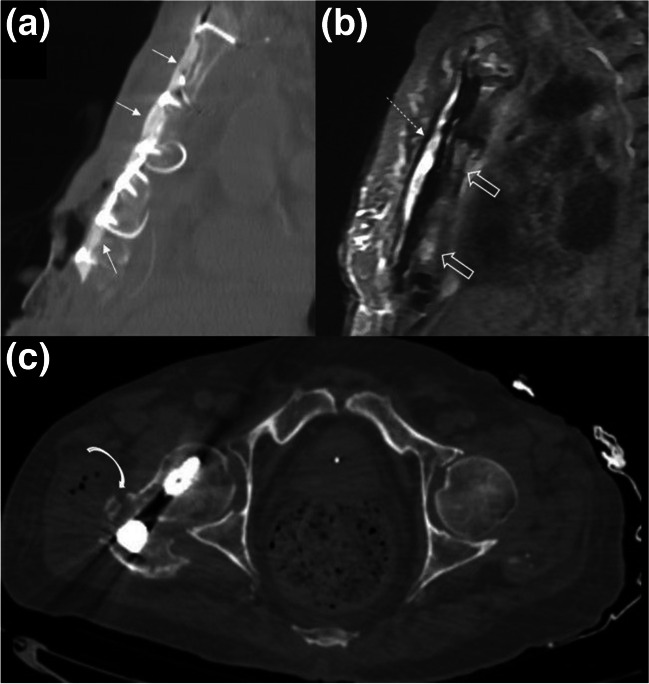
Sagittal reformatted CT (a) and multi-acquisition with variable-resonance image combination (MAVRIC) proton density fluid MR image (GE Healthcare) (b) of a patient with sternal osteomyelitis and presternal soft tissue infection, 3 months after cardiac surgery, are shown. The soft tissue defect at the lower quadrant of sternal surface is appreciated on CT image. Antibiotic-eluding bone cement and metallic wires were used to reconstruct the sternal defect which can be appreciated on CT image (arrows). Using a metal artifact reduction techniques on MRI, collection overlying the bone cement (dashed arrows) and edema-like signal changes in the presternal soft tissues can be appreciated. Note the relatively insignificant susceptibility artifacts due to the metallic wires on this sequence. Underlying corpus sterni shows high signal intensity areas (open arrows) which suggest sternal osteomyelitis. Axial CT image (c) in bone window in another patient with infected right hip prosthesis demonstrates periarticular collection with air bubbles and bone fragmentation at the anterior aspect of greater trochanter (curved arrow)
